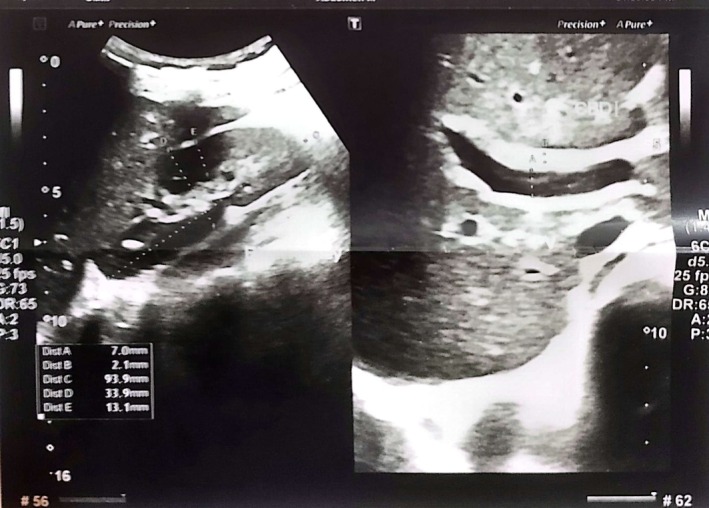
Ultrasound image showing gallbladder wall thickening without calculi or sludge. The wall measures 12.7 mm in thickness, consistent with acute cholecystitis secondary to viral hepatitis.
